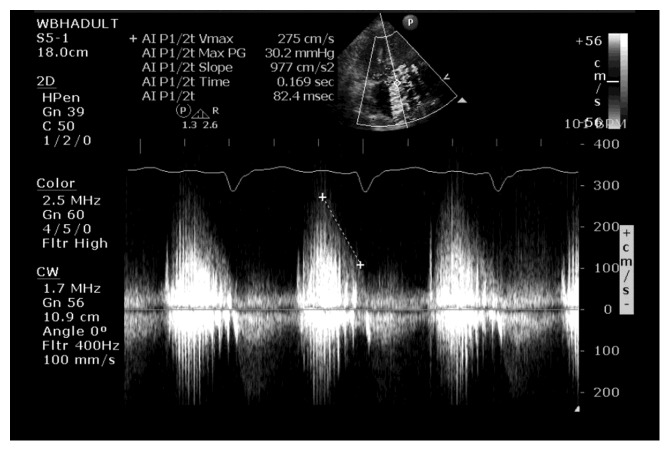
Transaortic Doppler demonstrating dense aortic regurgitation signal with markedly reduced pressure half time, suggestive of severe aortic insufficiency.
